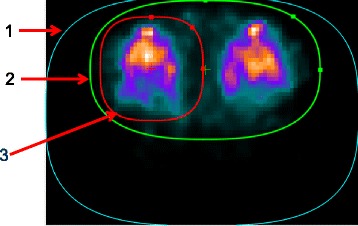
Planning a ^99m^Tc MDP SPECT study of the knees on the Valiance X12 prototype. The planning contours are overlaid on top of a transaxial slice of a low-resolution preview knee study. (1) Detector proximity contour (blue). Detectors are planned to encircle this contour. It is noteworthy that closer proximity for this patient was not possible due to the patient bed and cushions underneath the bent knees and bed straps on both sides. (2) Total activity contour (green), used to instruct the system to focus the detectors only within this ROI. (3) ROI contour (red). Used to instruct the system to dedicate more acquisition time when the detectors are focusing at this region, in accordance with user preferences (typically 50–90%)
